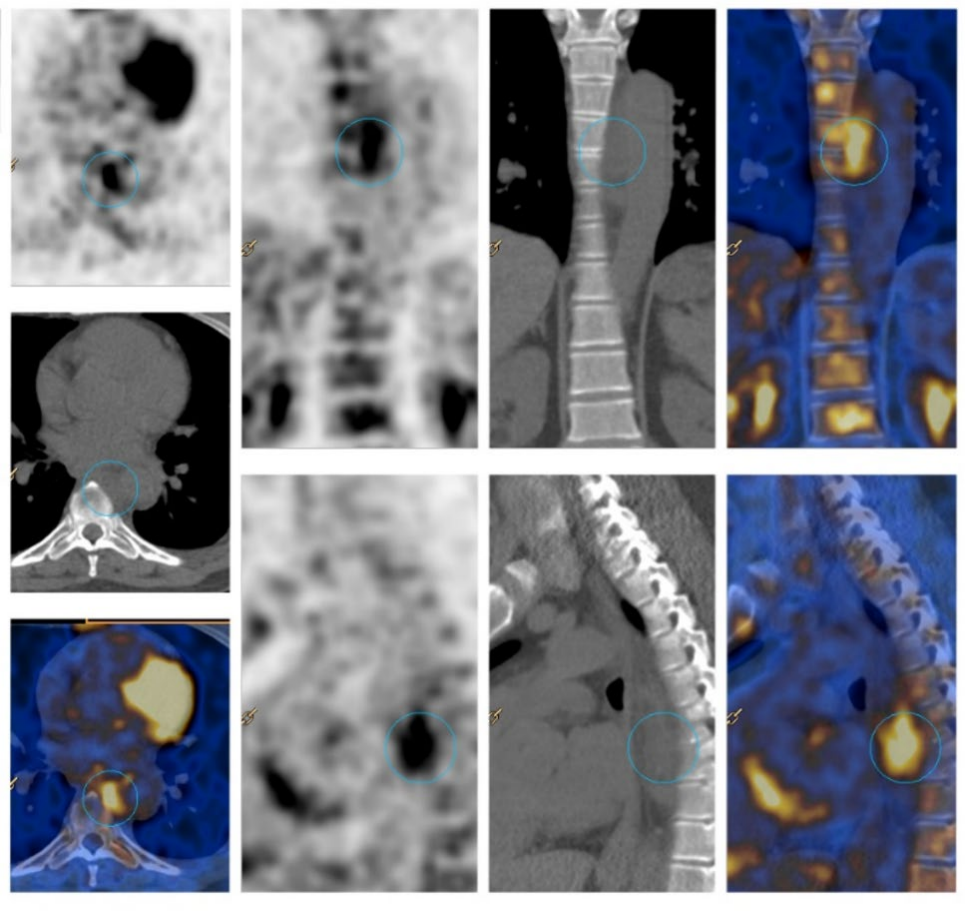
Case 8, 18-FDG–PET/CT scans of para-aortic infiltrative tissue at the level of the thoracic aorta and bone marrow hypermetabolism. *18-FDG* 18 fluorodeoxyglucose, *PET/CT* positron emitting tomography/computed tomography
